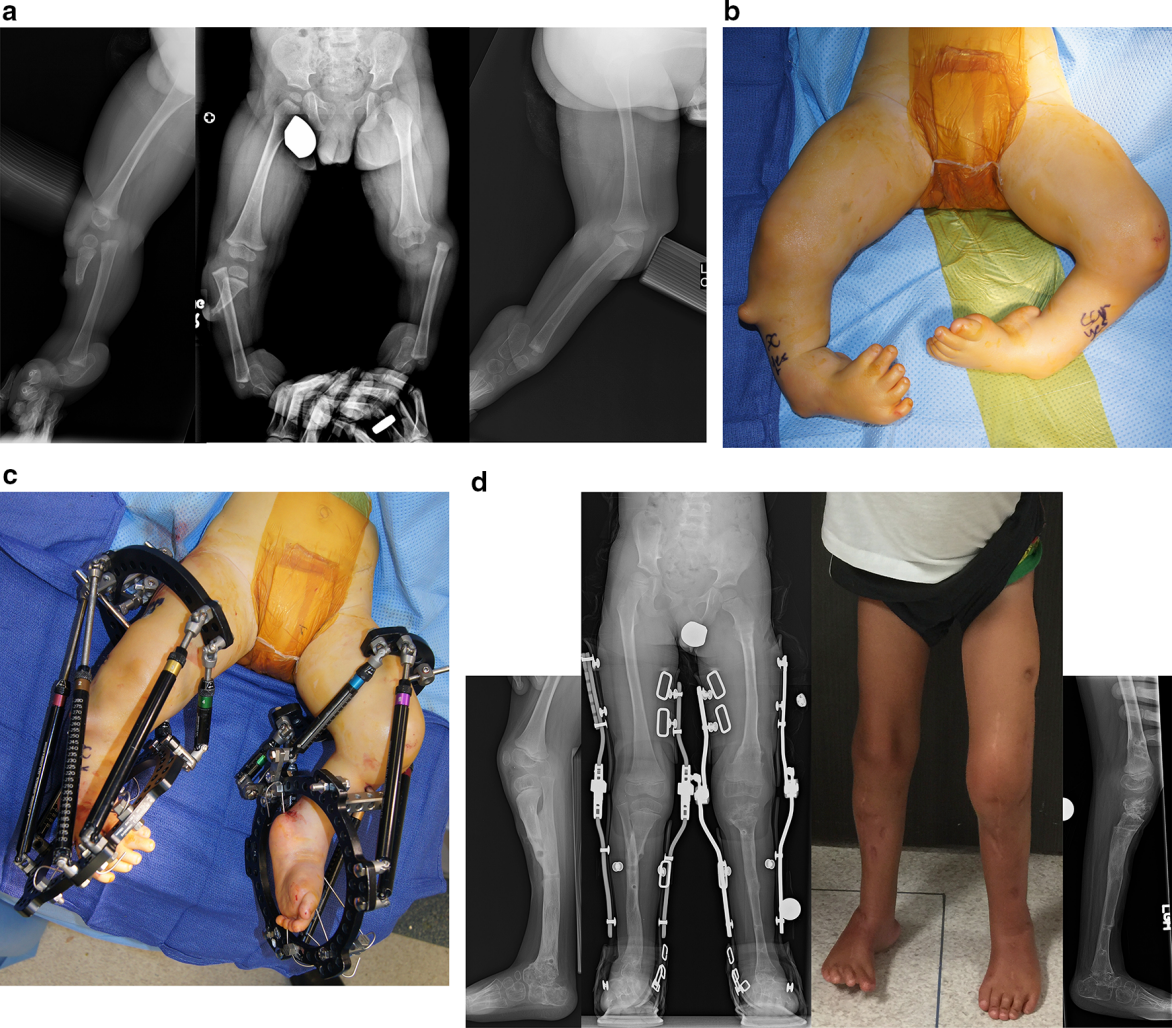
a Preoperative radiographs of a 13-month-old boy with bilateral tibial hemimelia; Paley type 4A tibial hemimelia on the *right* and Paley type 4B tibial hemimelia on the *left*. Both sides have severe equino-varus deformities of both feet. The proximal tibial remnant on the *right* is prominent under the skin. There is proximal migration of the fibula on both sides. There is an unossified proximal tibial cartilagenous anlage on the *left* side. b Clinical photograph showing the severe equino-varus feet. Note that the tip of the proximal tibial remnant has its own skin pouch. c Bilateral TSF devices were applied to both legs. The thigh rings are perpendicular to the femurs and the foot rings are parallel to the soles of both feet. This computer-dependent external fixator is programmed first to correct the deformities between the femur and fibula at the knee, including transporting the fibula distally. It is then reprogrammed to correct the foot deformity. No additional surgery is required to switch from knee to ankle correction. On the left leg the proximal fibular epiphysis was transported distally and brought under the tibial epiphysis. d Standing AP photograph (*center right*) and radiograph (*center left*) of both lower limbs at age 5 years, showing that the legs are well aligned. Lateral radiographs of right leg (*left*) and left leg (*right*) showing the feet are fused at the ankle in a plantigrade position. Both distal fibular physes are closed despite best efforts to preserve them. The right foot has some adductus supination deformity. The patient is shown after lengthening of both lower limbs was completed. The left proximal tibial epiphysis is ossified and fused to the proximal fibular epiphysis. The unossified proximal tibial epiphysis was ossified by insertion of BMP2 into drill holes in the cartilage of the tibial anlage. The ossification of the tibial epiphysis and fusion to the fibula occurred within 3 months. Note, there is preservation of the proximal physis of the fibula. On the *right* the fibula was transferred to the tibia. It has auto-bridged across to the proximal fibula. Initially, knee–ankle–foot–orthotic (KAFO) braces were used. The knee was stable enough to discontinue these after these radiographs
